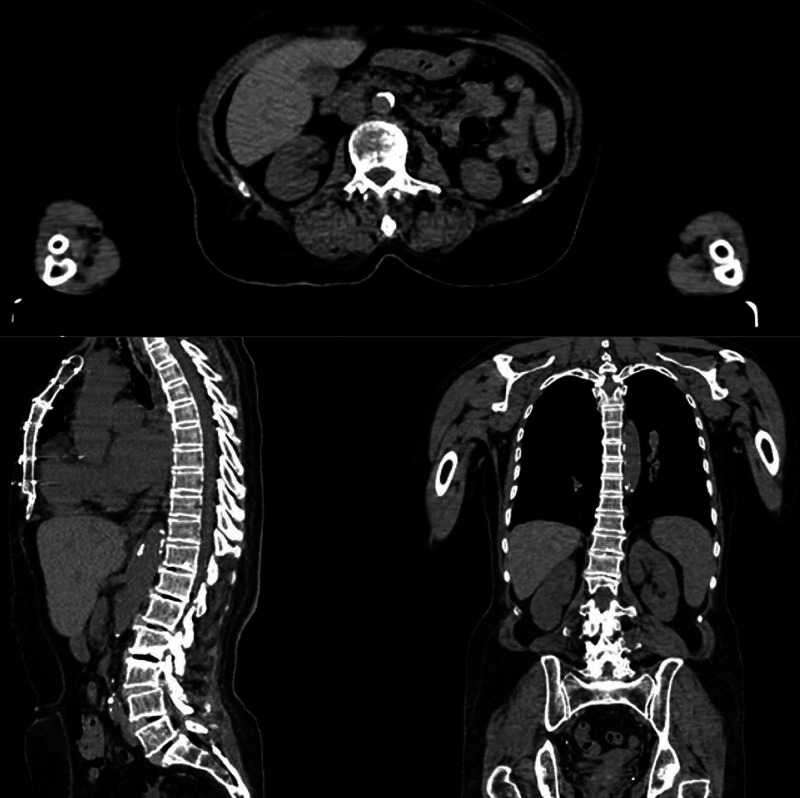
CT images for three-dimensional modeling of a patient with rotoscoliosis CT: computed tomography
